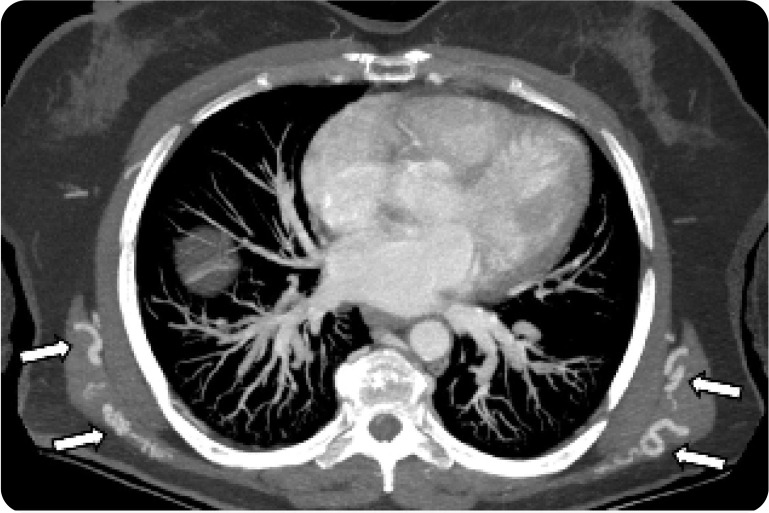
Axial CT Chest MIP reformat showing large collateral circulation arising from intercostal arteries coursing through the posterior chest wall (white arrows). These collaterals will go and reconstitute/supply the upper extremities circulation (not shown).
